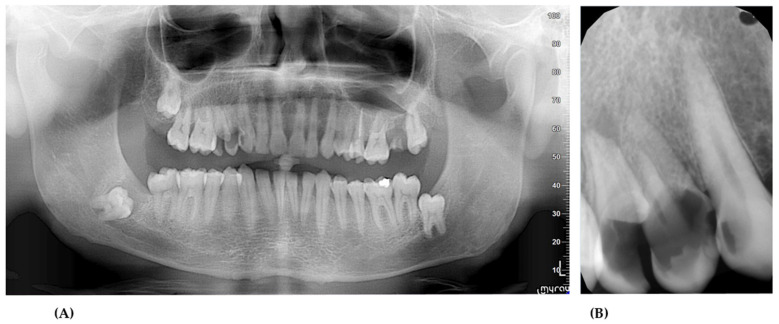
Orthopantomography (A) and radiovisiography (B) before surgical treatment.
